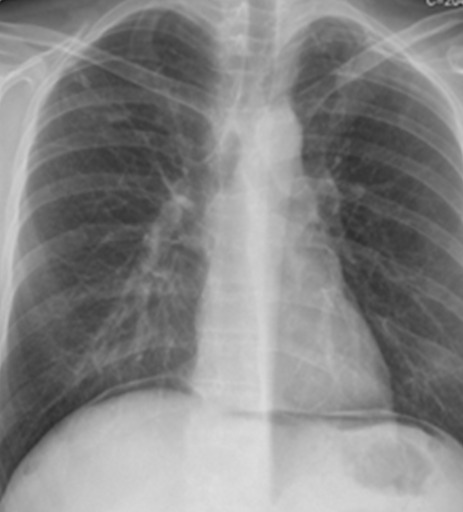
Thoracic radiography with pneumoperitoneum
